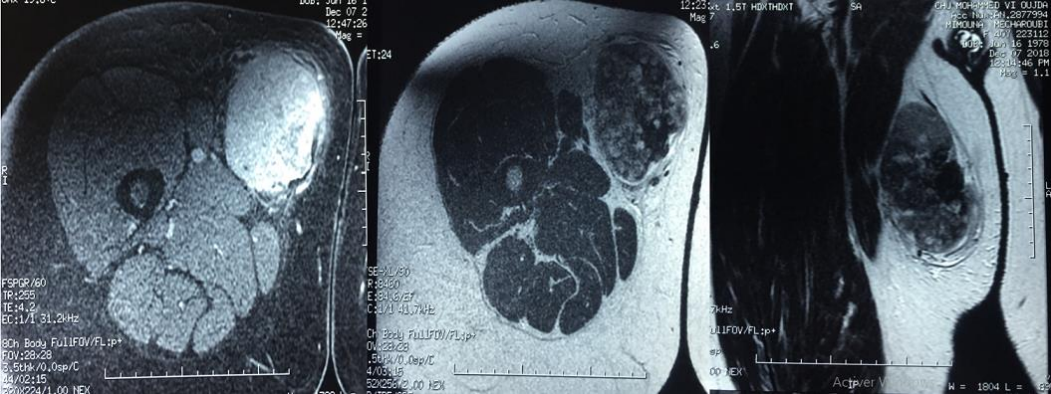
MRI appearance of the mass
